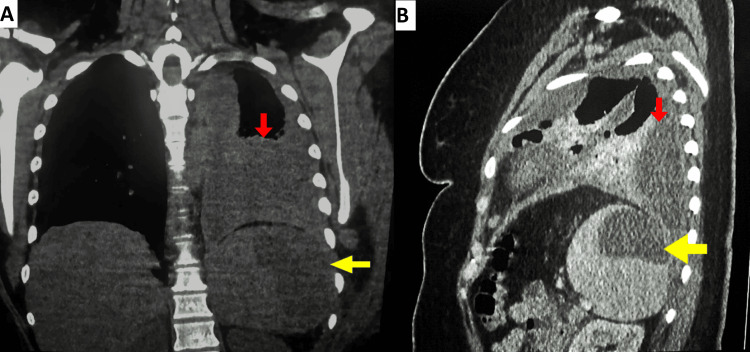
Contrast-enhanced CT in the coronal plane (A) and sagittal plane (B) revealed an expansion of the splenic hematoma (yellow arrows) and an increase in the left-sided pleural effusion (red arrows).
